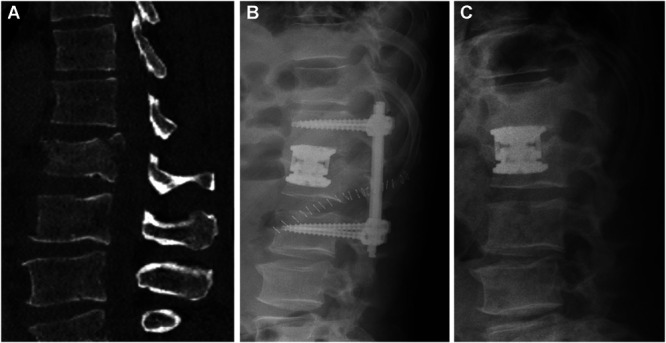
Case example of a surgically treated (incomplete, *Arbeitsgemeinschaft für Osteosynthesefragen* spine type A3) burst fracture of the 3rd lumbar vertebrae in a 39-year-old man. A, Initial computed tomography scan showing the fractured vertebrae involving the upper endplate. B, Postoperative x-ray after implantation of an expandable cage. C, X-ray after dorsal implant removal showing the preserved spinal alignment.
